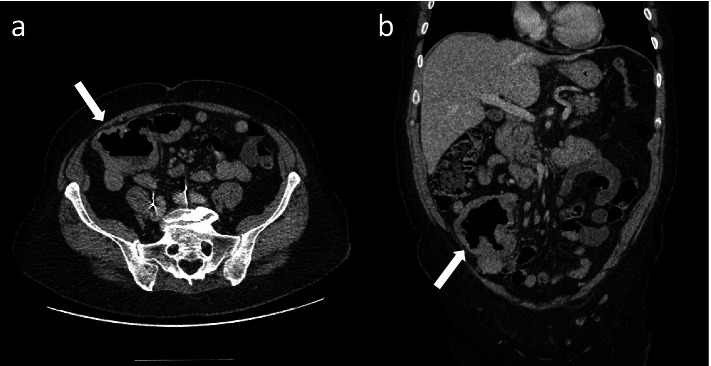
a Axial and b coronal CT images of the abdomen and pelvis with a large necrotic pelvic mass arising from the mid ileum with aneurysmal dilatation (white arrows)
